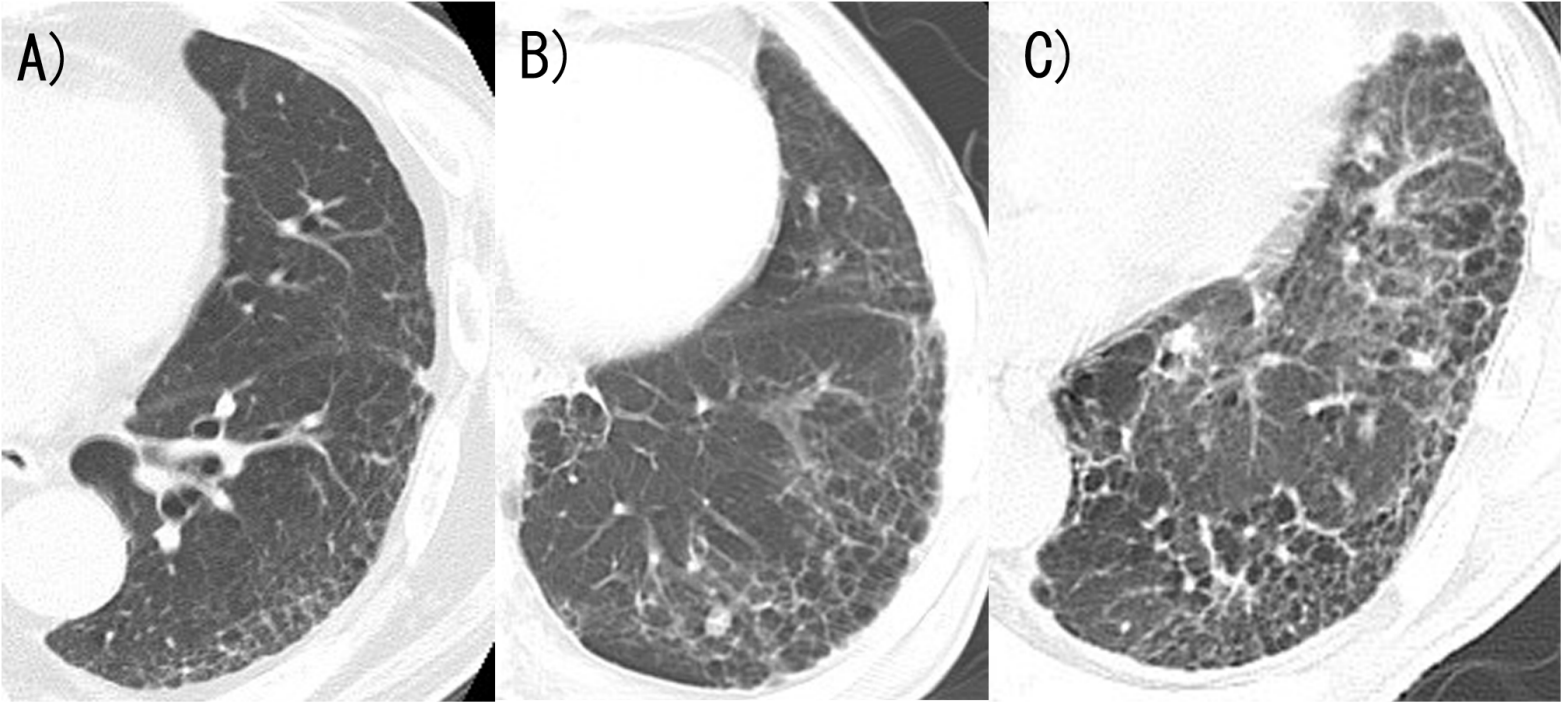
Representative chest computed tomography image of the area used for assessing the presence of preexisting interstitial lung disease. A), B), and C) were scored as 0–10, 10–25, and 25–40%, respectively.
